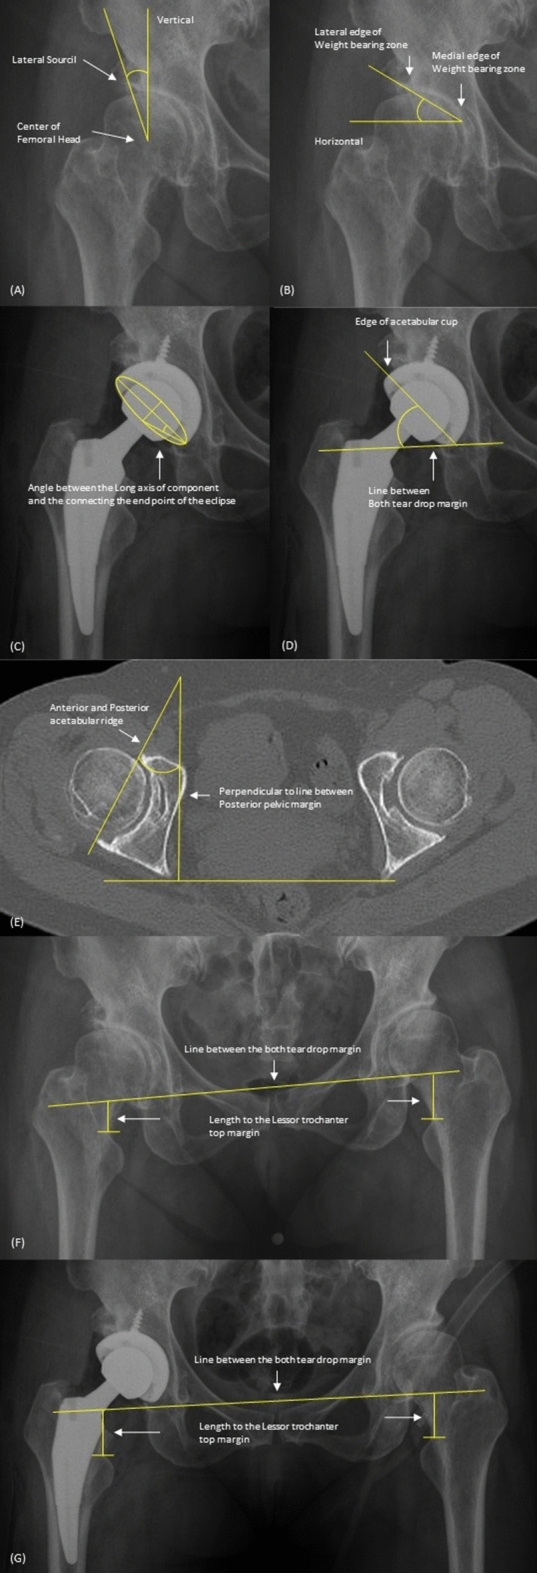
Radiographic measurement for preoperative evaluation and postoperative outcomes. (A) Mesurement of Lateral center edge angle (LCEA), (B) Mesurement of Tönnis angle, (C) Mesurement of angle for Liaw’s method, (D) Mesurement of cup inclination, (E) Mesurement of acetabular anteversion of CT, (F) Mesurement of preoperative Leg length discrepancy (LLD), (G) Mesurement of postoperative LLD.
